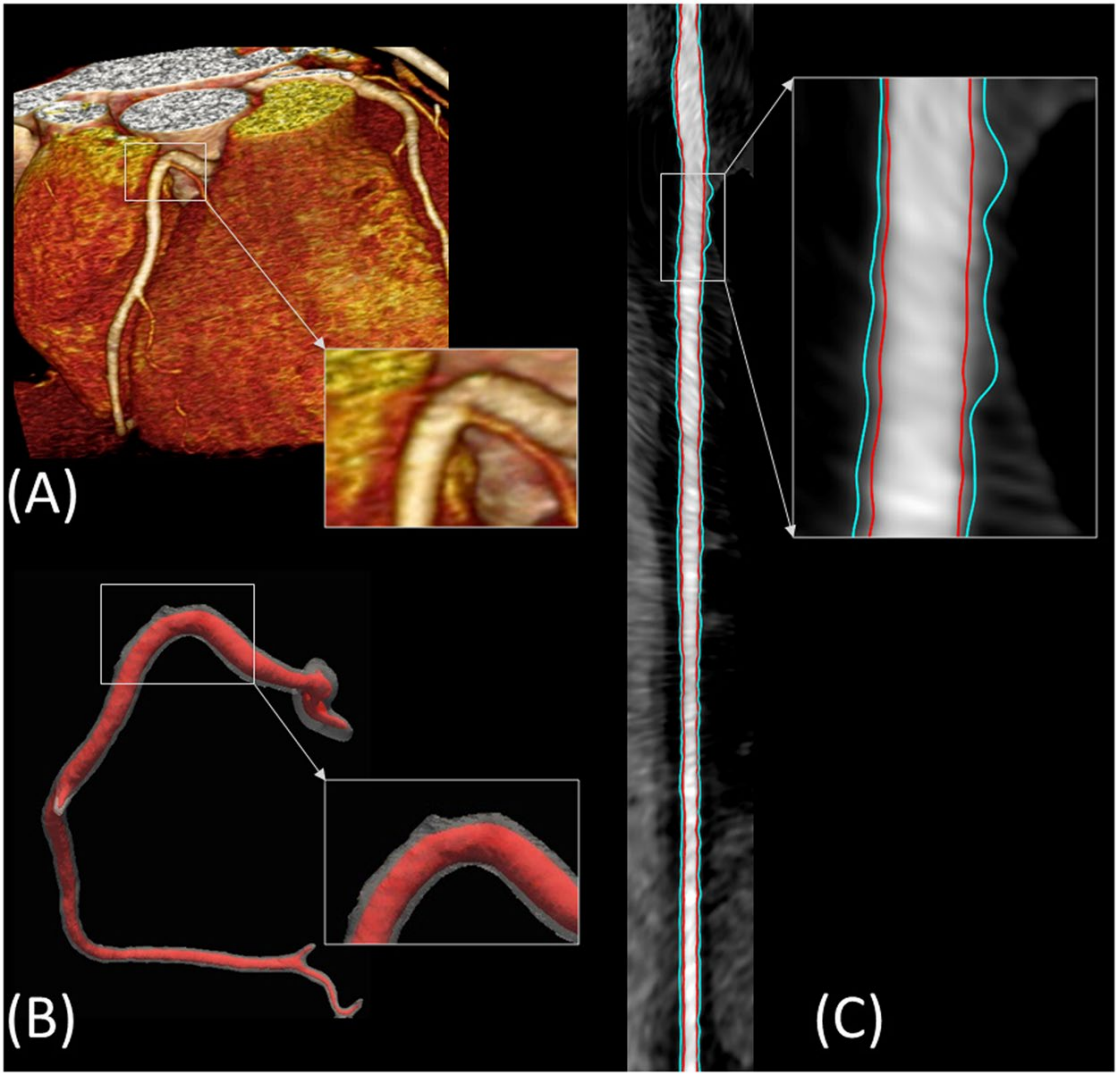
Visualization of framework segmentation. (A) The input 3D coronary CTA dataset. (B) Segmented lumen (red) and wall (gray). (C) Straightened vessel demonstrating the delineated inner and outer boundaries of the vessel in red and blue, respectively.
